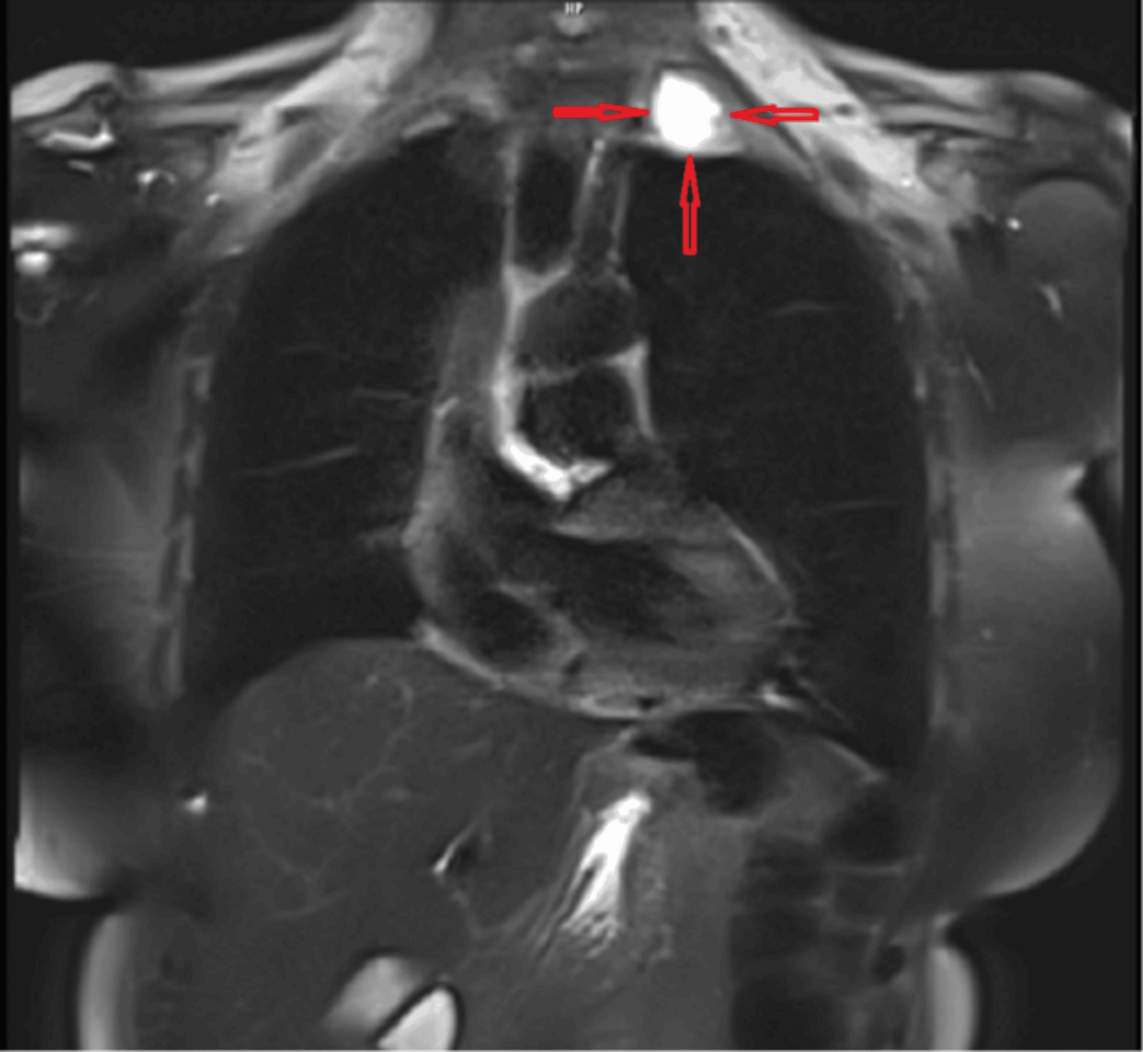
Coronal section of magnetic resonance imaging showing the location and appearance of the lesion.
